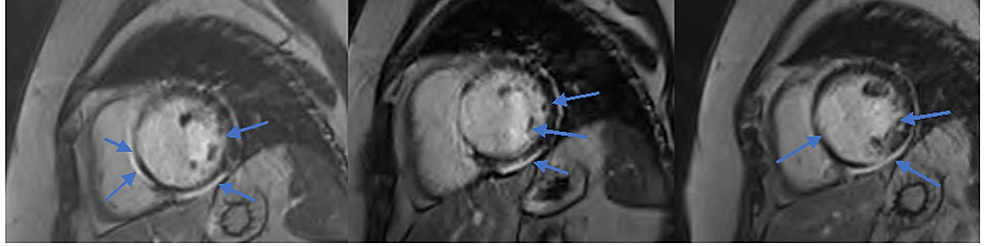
Post-contrast cardiac MRI revealing fibrosis from the base of the left ventricle to the right ventricular septum.
